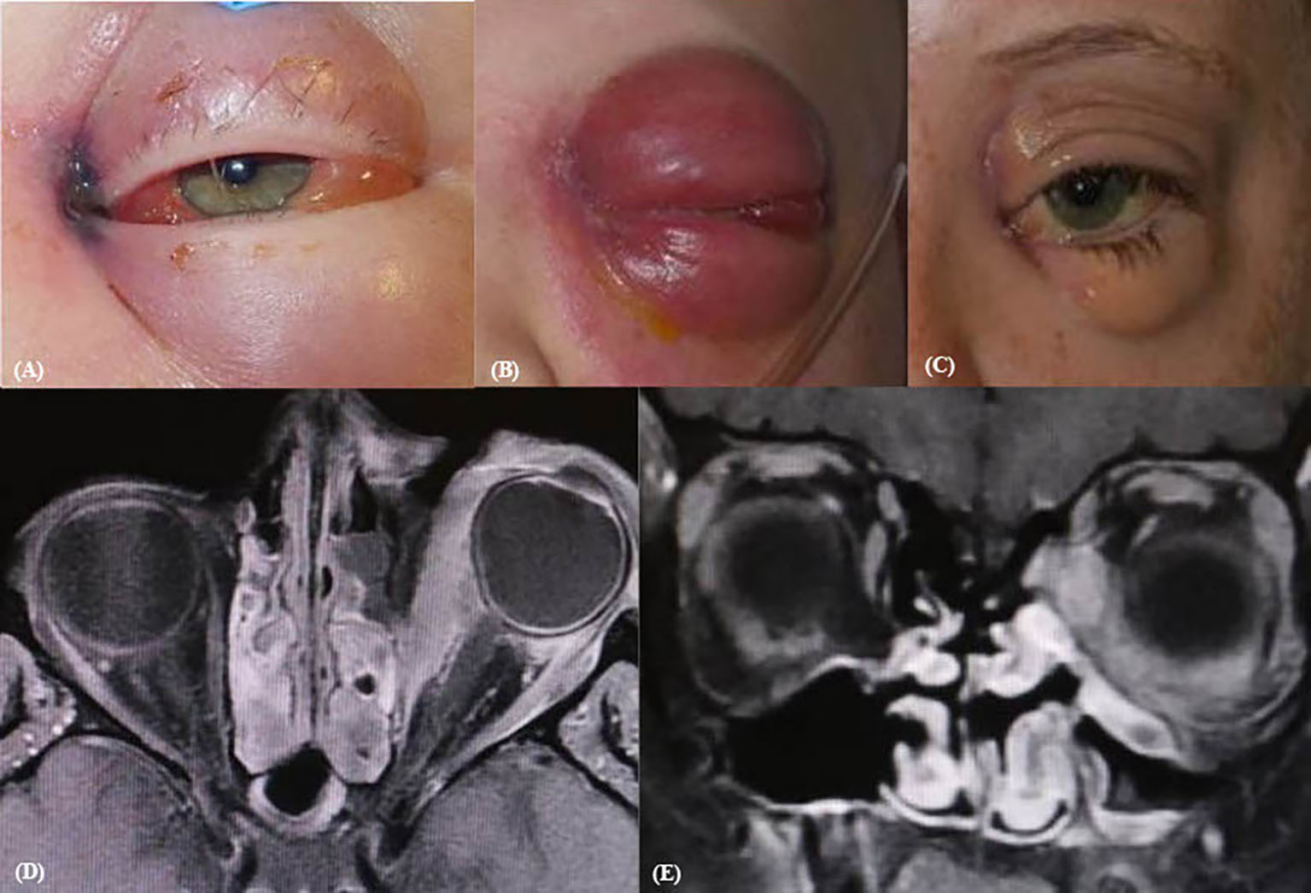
Pre- and post-operative imaging of case 3. Initial presentation pre-op (A), clinical progression (B), and post-reconstruction (C) gross images. Transverse (D) and coronal (E) CT orbit demonstrate visible peri-orbital enhancement and proptosis.
